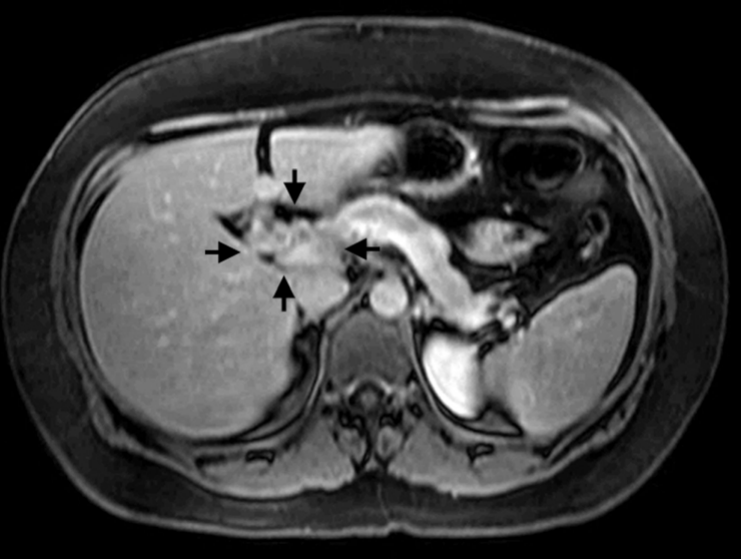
Axial T1, fat-suppressed, portal venous phase MRI performed prior to resection. Arrows define the boundaries of the cholangiocarcinoma.
